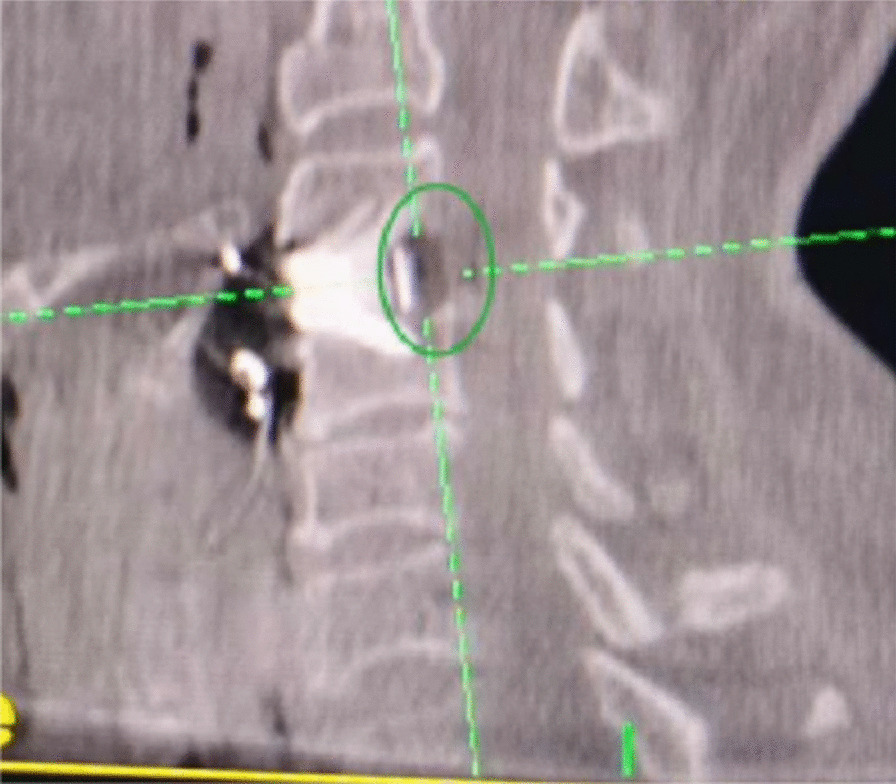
Postoperative CT showed that Complete bone debridement and adequate spinal cord decompression
